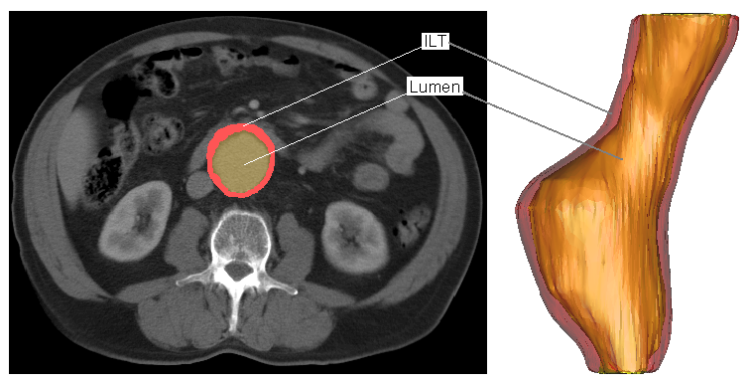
Typical CT scan and 3D reconstruction of patient suffering from an AAA. On the left shows a typical CT scan after segmentation using Mimics v10.0, with the ILT (red) and lumen (yellow) clearly distinguishable. 3D reconstruction of examined AAA is shown on right.
